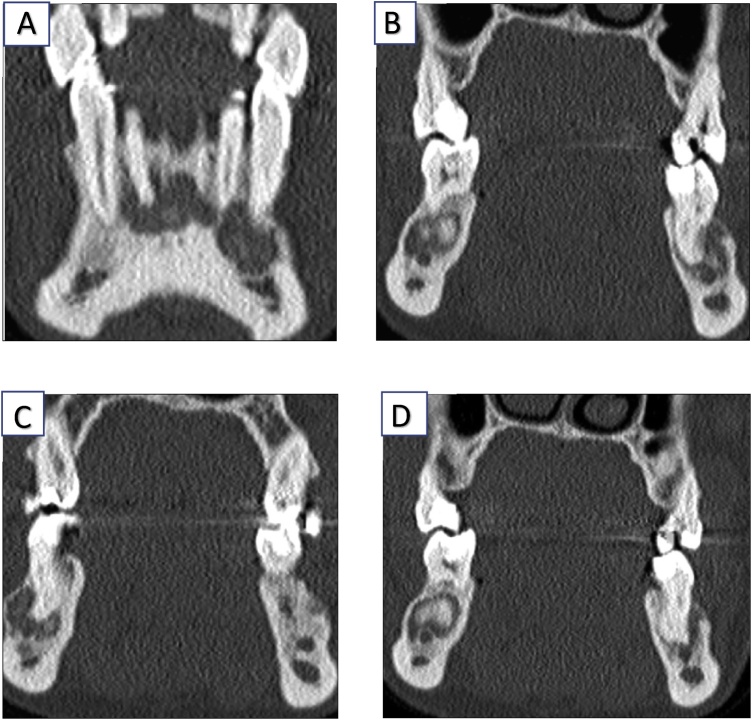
A–D are the computed tomography images, which shows us different layers of the CT in the horizontal cut.
